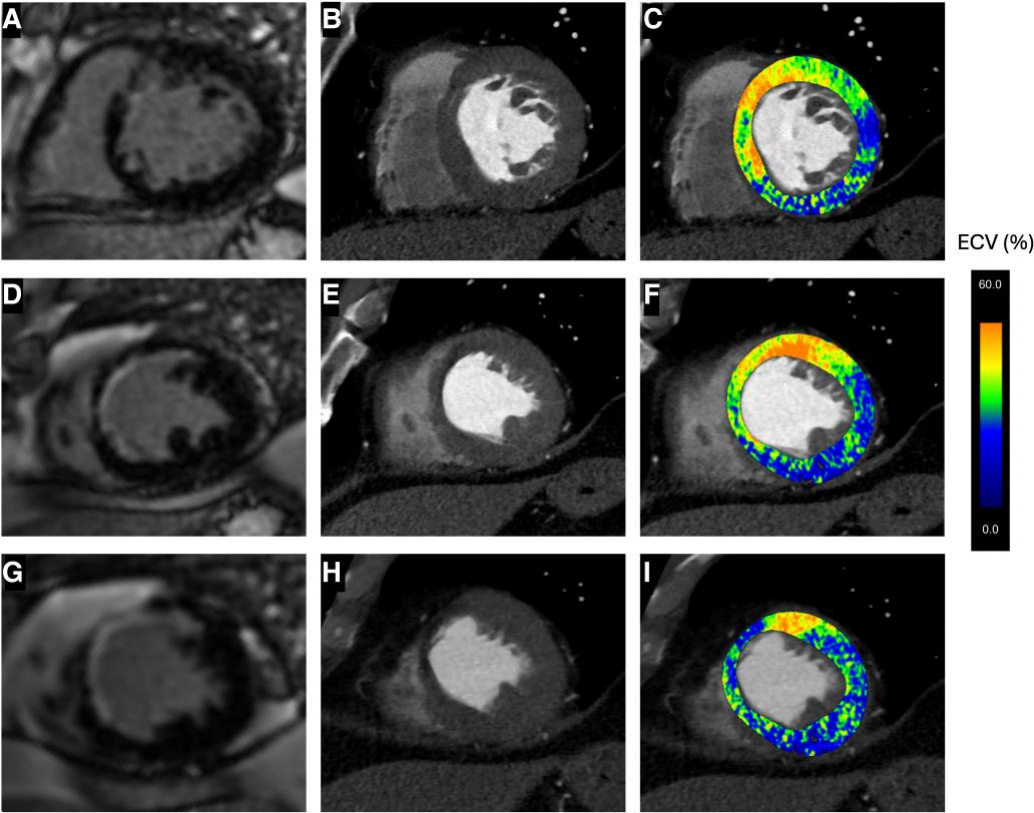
Multimodal imaging of lipomatous metaplasia (LM) – short axis planes. Panels A–I are arranged in rows corresponding to basal (*A–C*), mid (*D–F*), and apical (*G–I*) left ventricular segments. The left column (*A*, *D*, *G*) shows short-axis CMR images of late gadolinium enhancement, the middle column (*B*, *E*, *H*) shows corresponding CT images, and the right column (*C*, *F*, *I*) displays colour-coded ECV values overlayed on the CT image. Abbreviations: CMR – Cardiac Magnetic Resonance, CT – Computed Tomography, ECV – Extracellular Volume.
